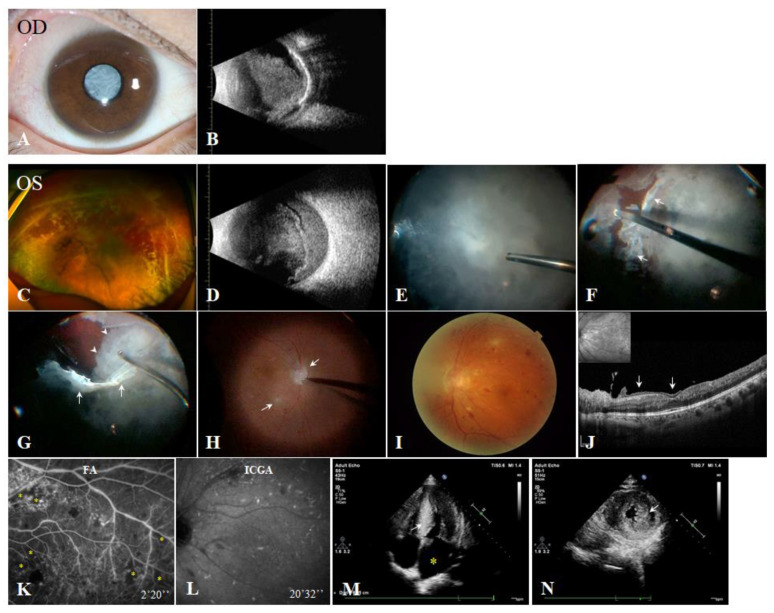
Case 2. (A) Anterior segment photograph of the right eye. (B) B-scan ultrasound image of the right eye, showing vitreous opacities and calcification of the eyeball wall. (C) Preoperative fundus photograph of the left eye, showing vitreous haze. (D) B-scan ultrasound image of the left eye, showing opacities in the vitreous cavity and preretinal space. (E–H) Intraoperative snapshot images showed cotton wool-like opacities (E), dense posterior hyaloids (F,G, white arrows), velvet deposits in the preretinal space (G, white arrowheads) and white deposits adhered to the optic disc and retina (H, white arrows). (I) Postoperative fundus photograph of the left eye, showing multiple hemorrhagic spots. (J) Postoperative optical coherence tomography image, showing deposits adhering to the surface of the retina and blurred retinal structures. (K) Fluorescein angiography of the left eye showed multiple areas of telangiectasis and nonperfusion. (L) Indocyanine green angiography of the left eye, showing hypercyanescence along the choroid vessels. (M) Apical four-chamber view of the heart on echocardiography, indicating left atrial enlargement and thickening of the ventricular septum. (N) Parasternal short-axis view at the level of the mitral valve, indicating hypertrophy of the left ventricular walls.
